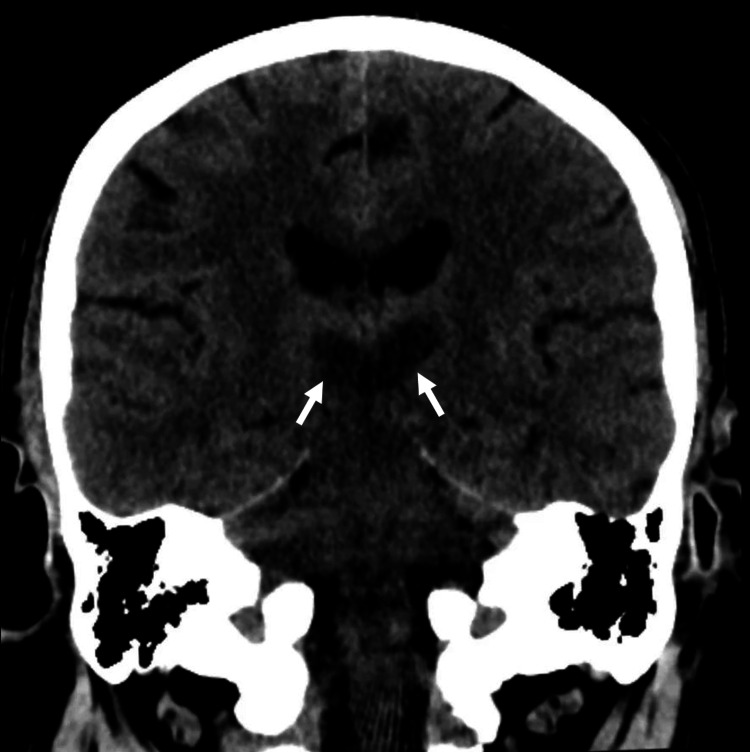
Coronal CT head image demonstrates bilateral thalamic hypodensity (arrows), which is highly suggestive of artery of Percheron infarction.
